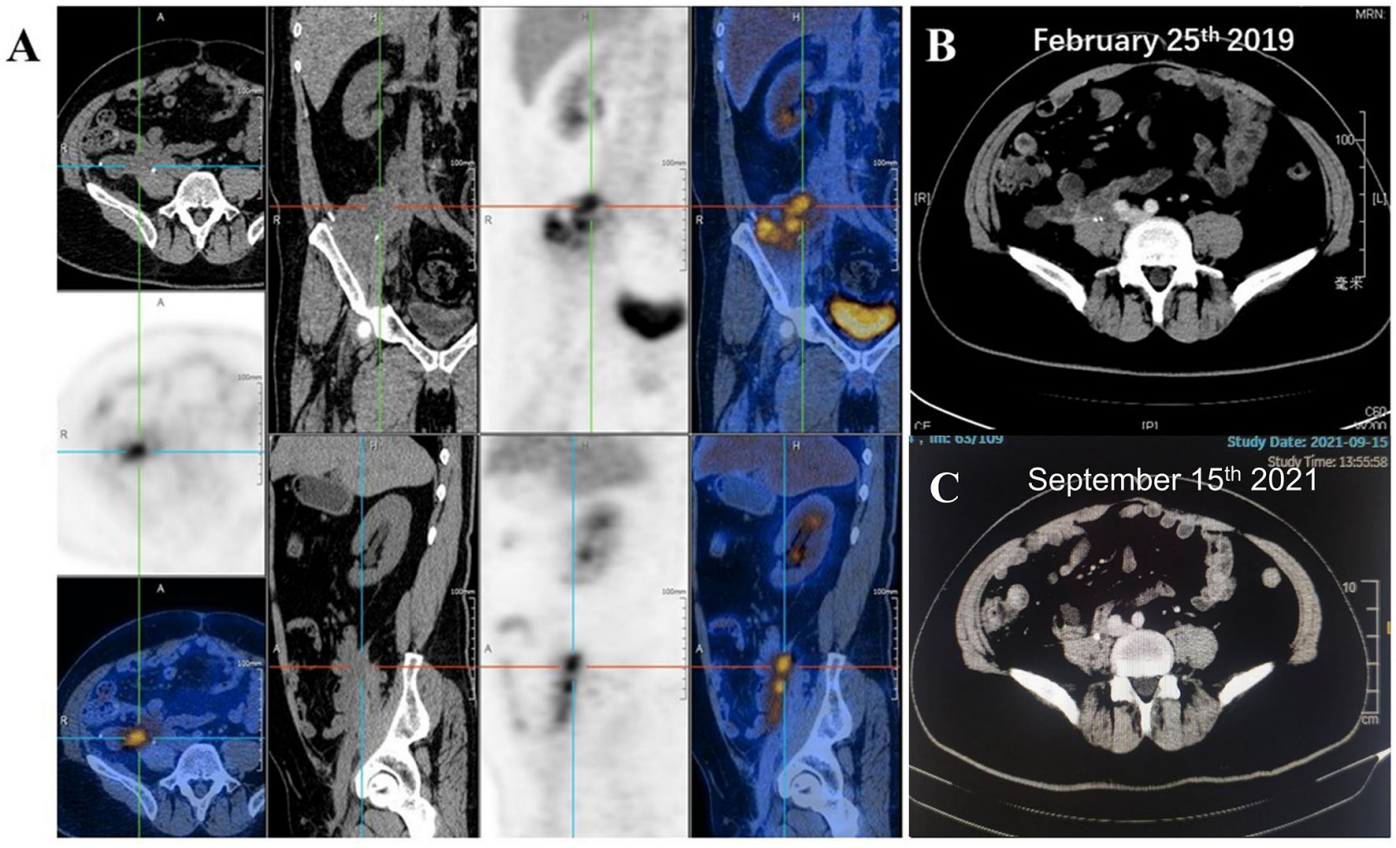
(A) Positron emission tomography/computed tomography (PET/CT) suggested a high local metabolic activity during the follow-up of March 2019. CT scans for the patient on (B) February 25 and (C) September 15, 2021.
